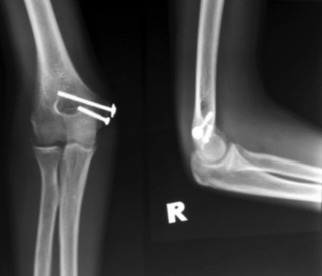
Radiographs showing united medial humerus epicondyle avulsion fracture.
